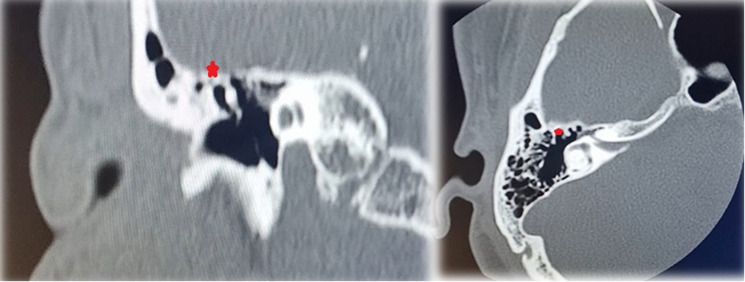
temporal bone CT scan: coronal view threw the anterior portion of the temporal bone on the right, and axial view threw the superior portion of the temporal bone on the left of the figure, the two images show ossification between the malleus head and the tegmen tympani (asterixis)
